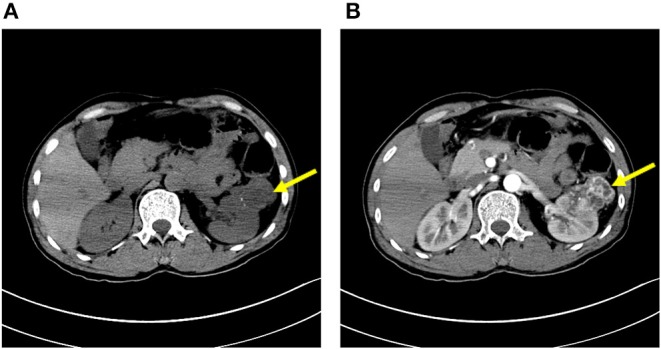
Plain CT (A) and contrast-enhanced scan (B) of the kidney. The arrow shows a mass of 5.5 × 5.0 × 4.0 cm on the left side.
